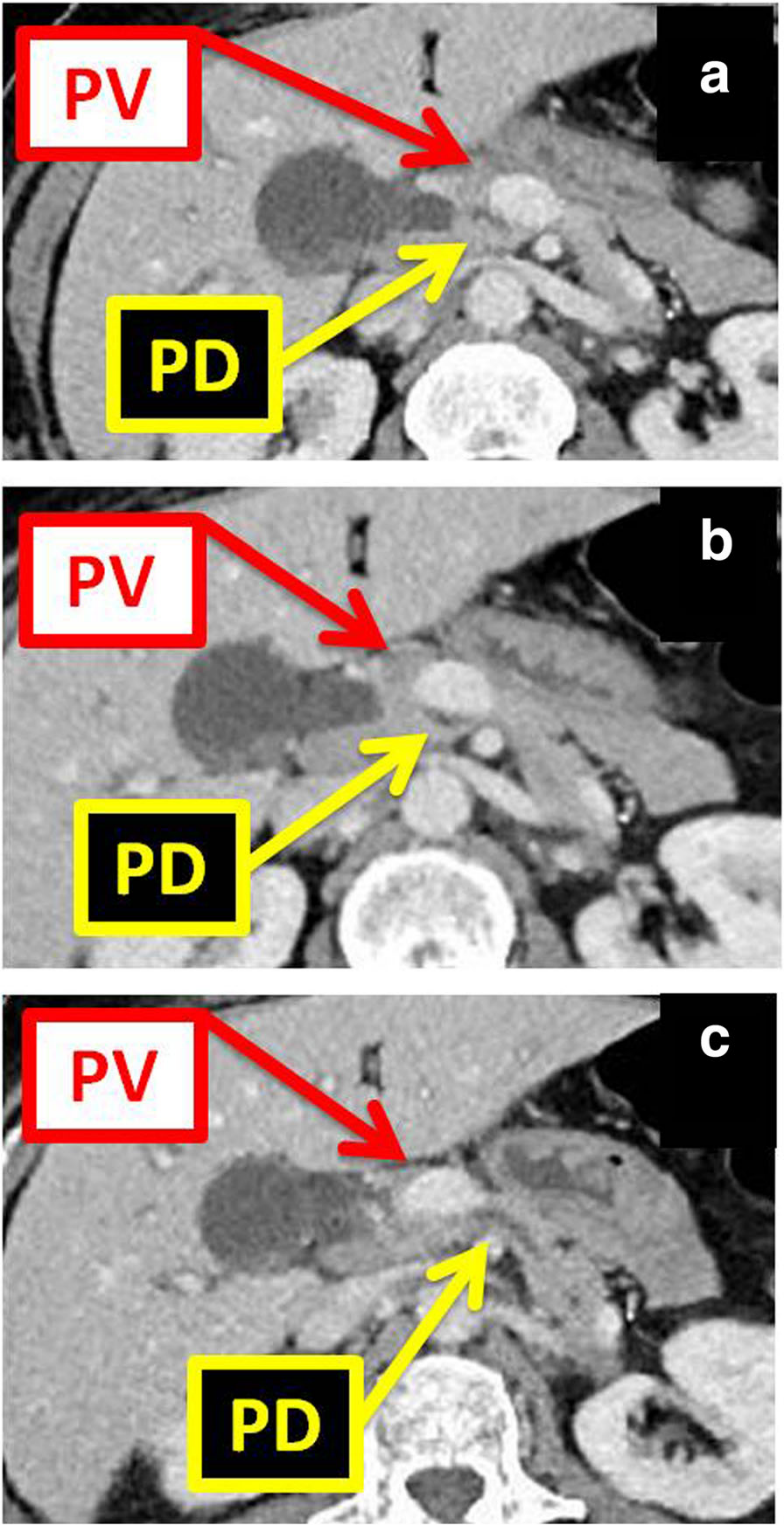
Axial images of computed tomography scan of the abdomen. The images show the portal vein in between the duodenum and the pancreatic duct. Three sequential cuts shown: a–c caudal to cephalad. *PD* pancreatic duct, *PV* portal vein
